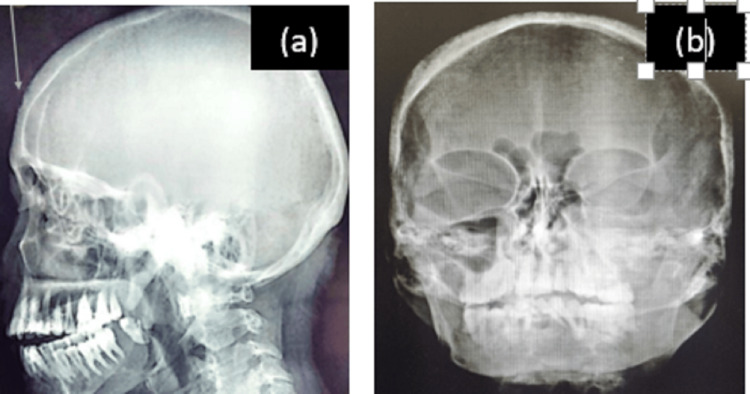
Skull radiograph (a) Lateral skull projection showing surface resorption of the skull, (b) PA skull projection showing salt and pepper appearance of the skull.
